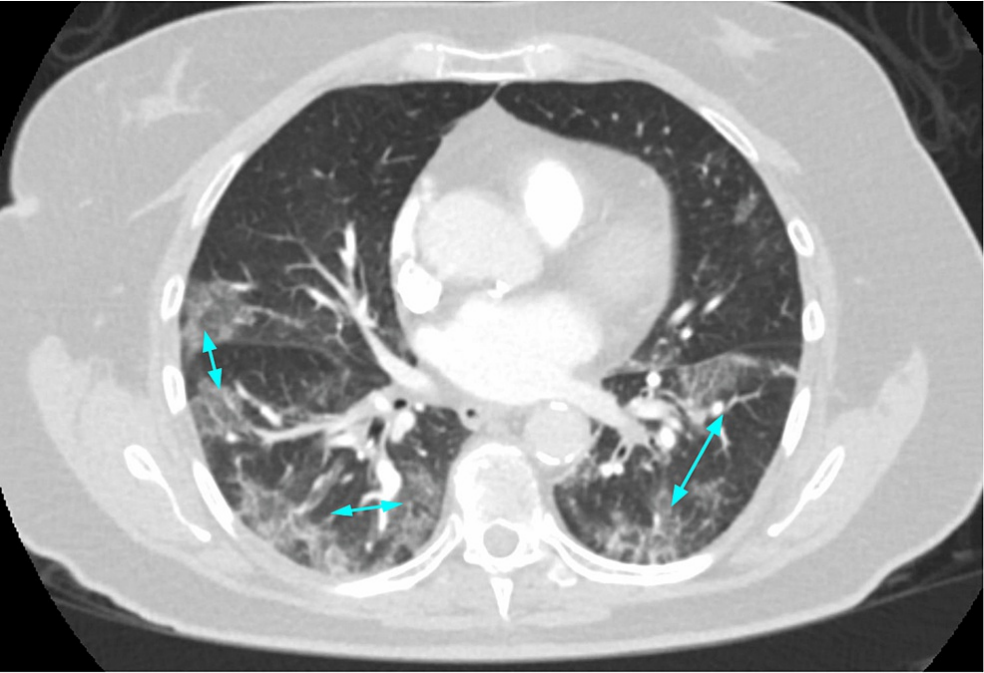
Typical peripheral, bilateral lower lung, ground-glass opacities (blue arrows) of COVID-19 pneumonia on CT.
